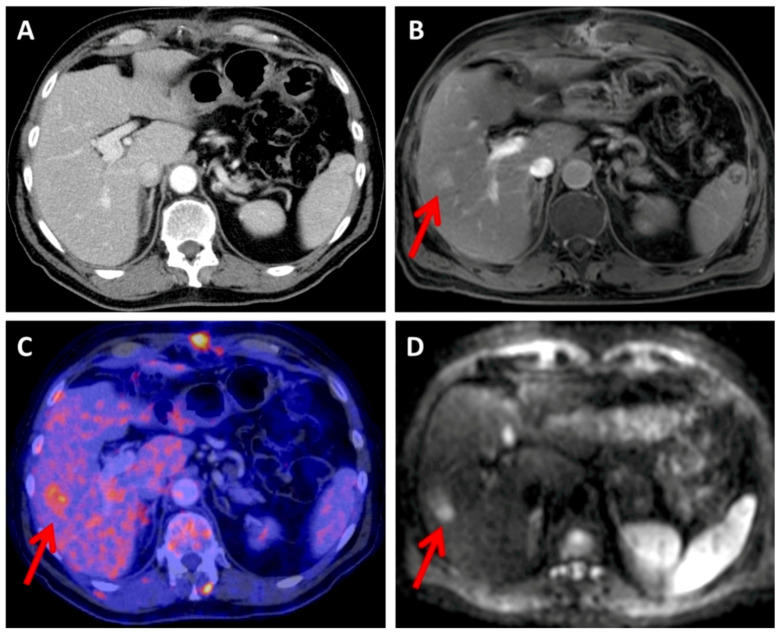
The melanoma patient had a metastatic hepatic lesion located in segment VIII (indicated by the red arrow), which is distinguishable in contrast-enhanced T1 VIBE (B) and DWI (D) images but is not visible in the CT scan (A). The lesion exhibits an elevated FDG uptake in the PET scan (C).
